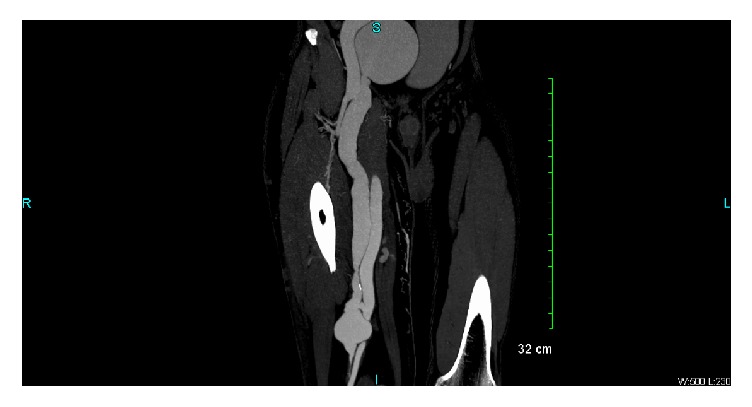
CT angiogram showing a superficial femoral arteriovenous fistula with arteriomegaly of the right external iliac artery.
